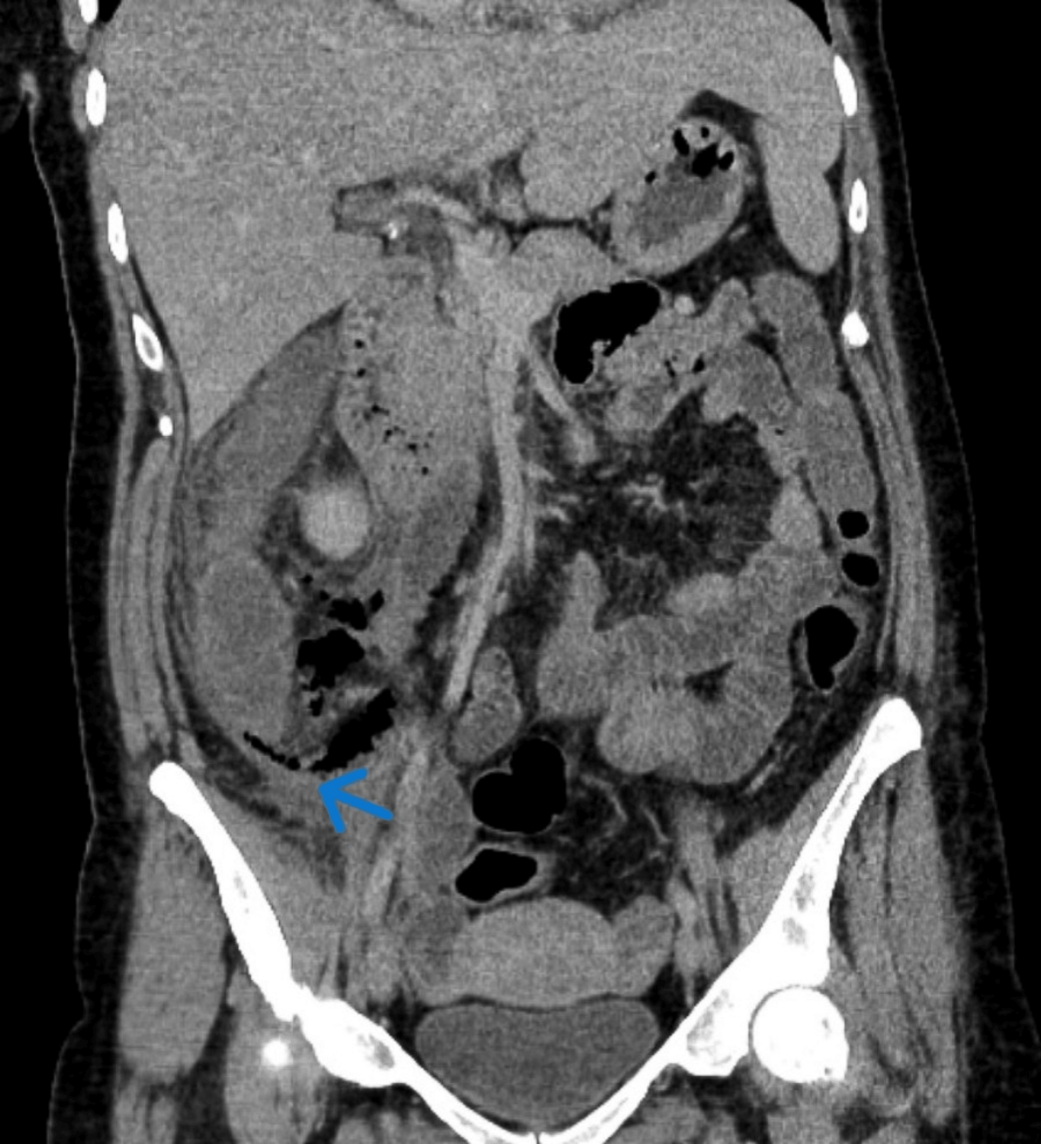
CT abdomen and pelvis (coronal section) demonstrating free air and fluids in the right side of the abdomen (blue arrow).
